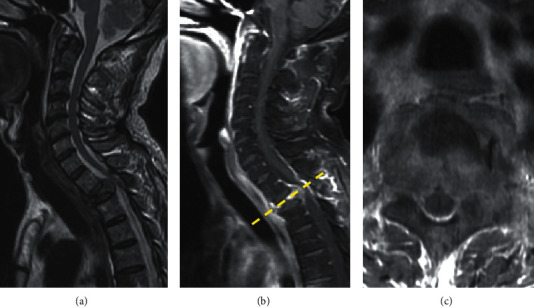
The patient presented spinal tumor at the T2 level with cord compression. (a) T2-weighted, (b) T1-weighted with a contrast sagittal view, and (c) T1-weighted with a contrast axial view.
